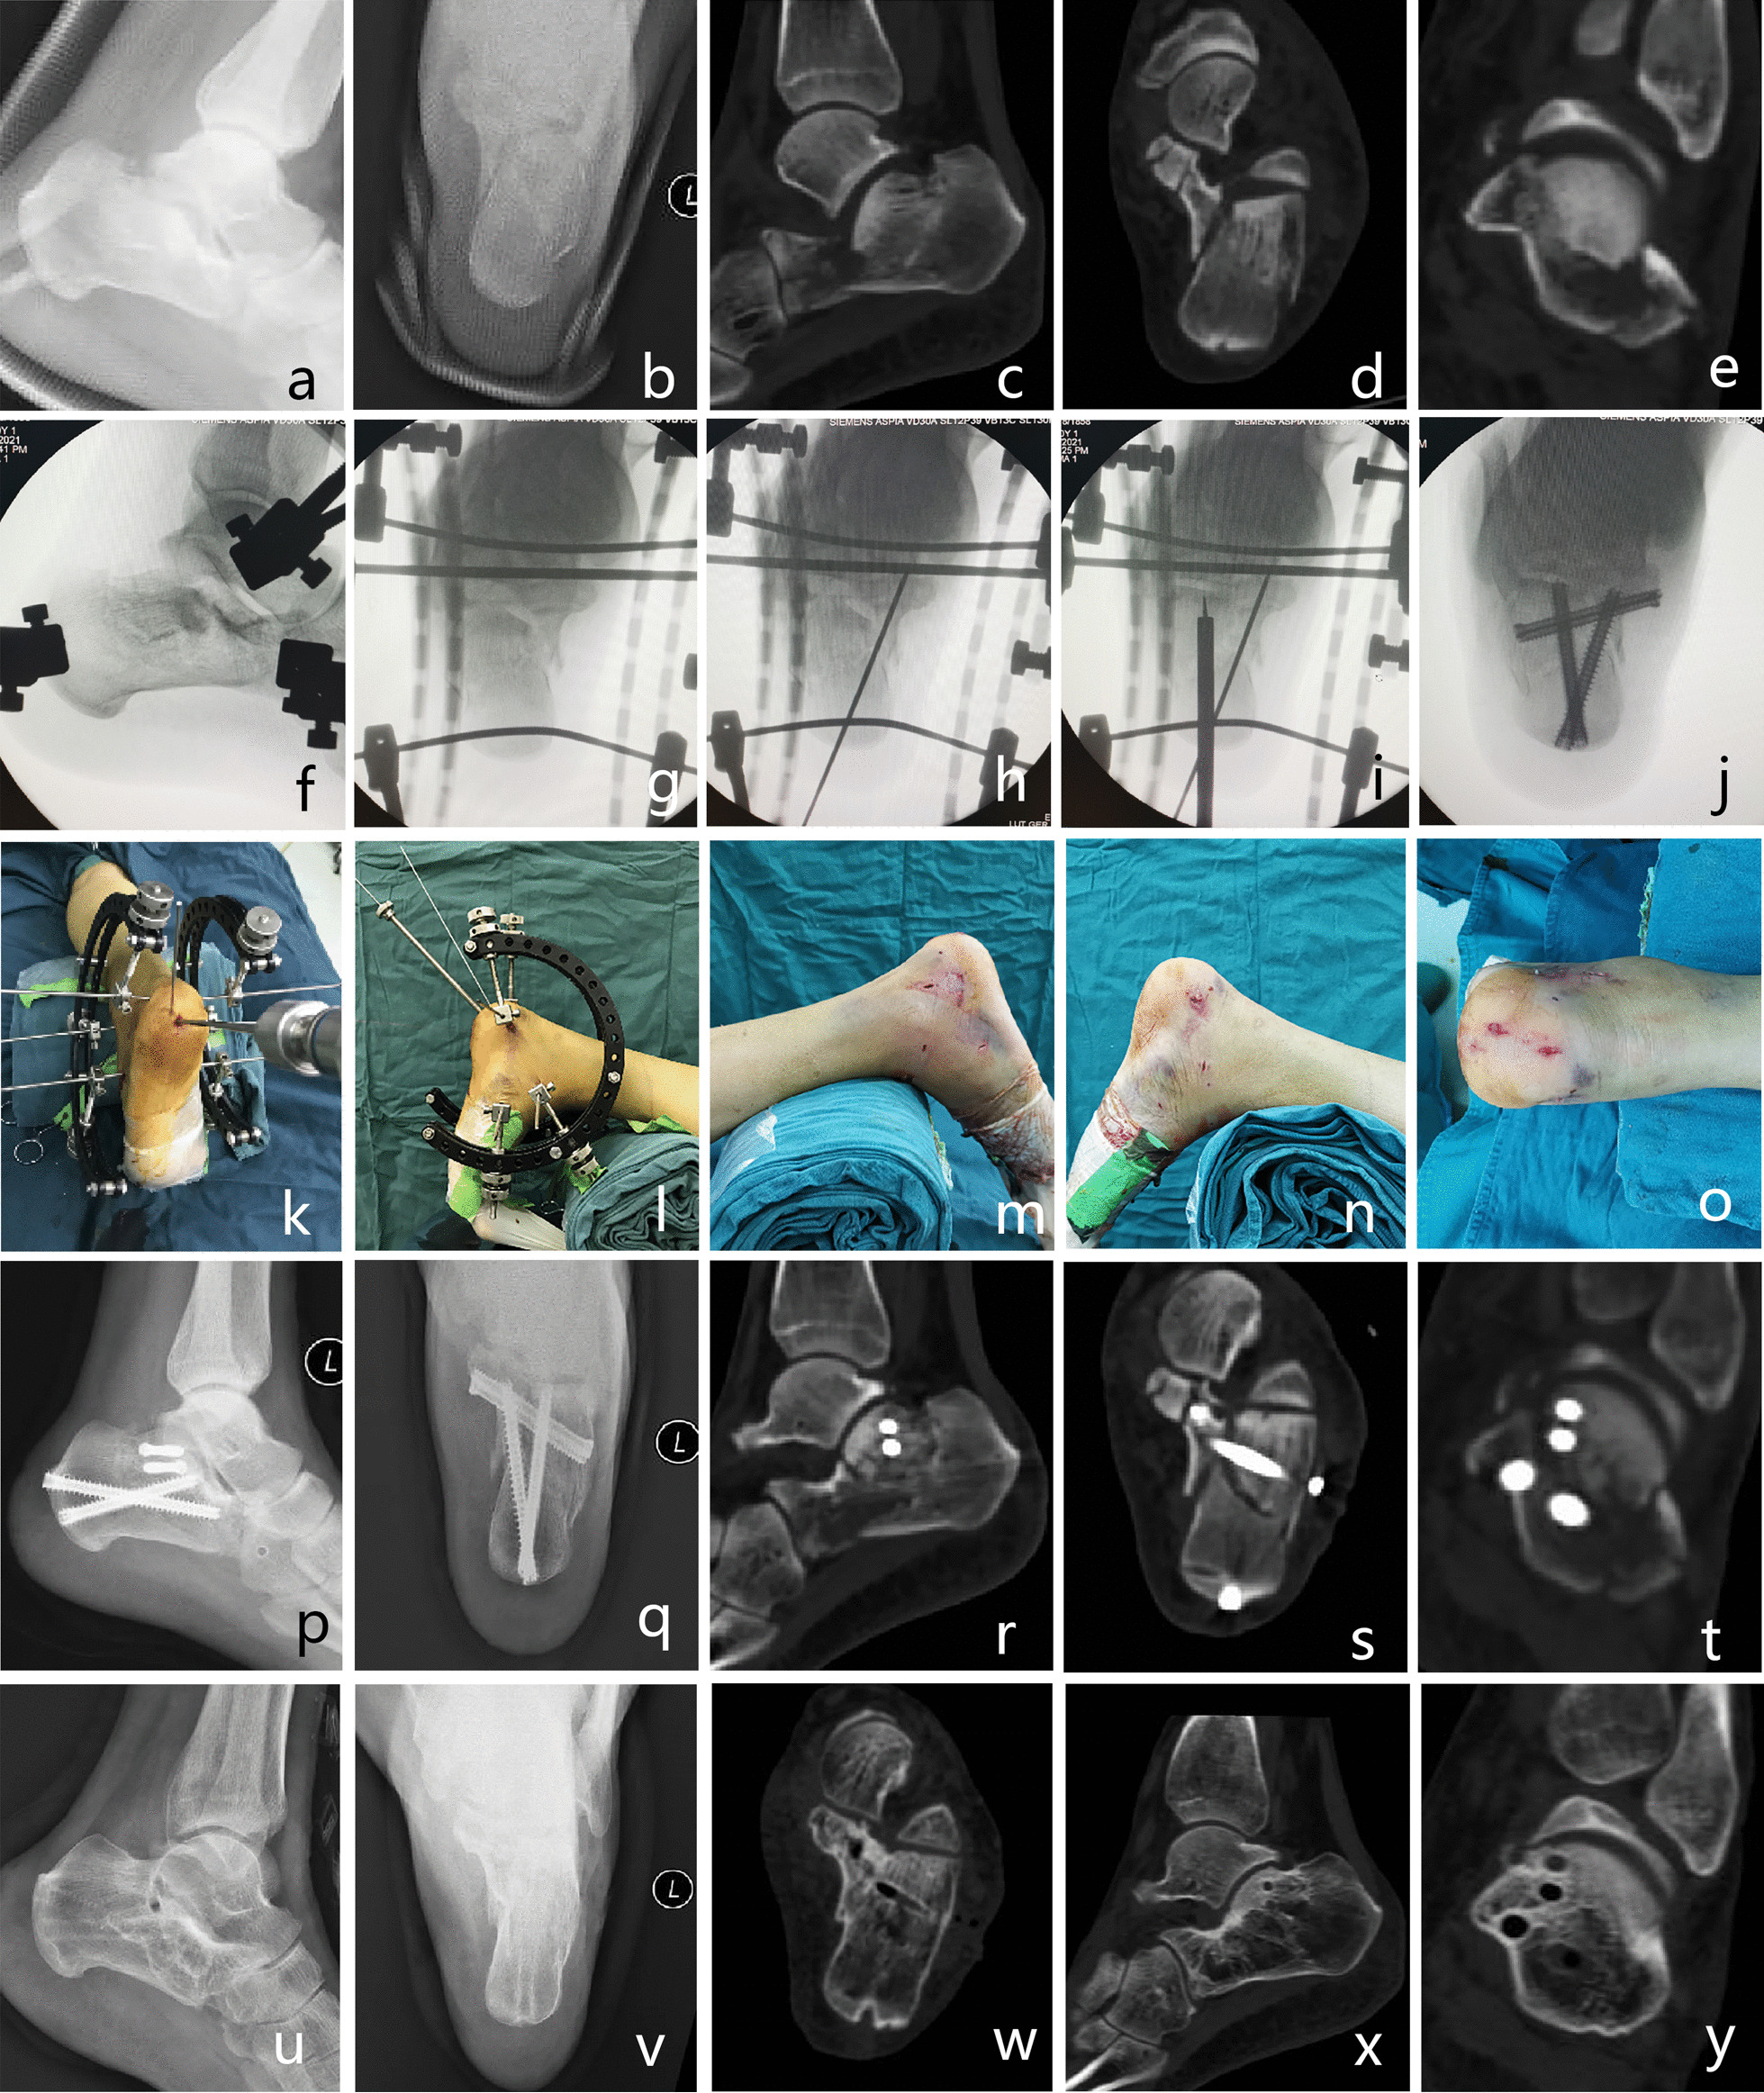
A 32-year-old male patient suffered left displaced intra-articular calcaneal fracture caused by a fall from a height, Essex-Lopresti classification (joint compression type), Sanders classification (II C). Preoperative lateral (a) and axial (b) X-rays, axial (c), sagittal (d) and coronal (e) CT views showed significantly decreased calcaneal height, Bohler angle and Gissanes angle, significantly increased calcaneal width and significantly collapse of the subtalar articular surface. Intraoperative fluoroscopy (f–j) showed the surgical procedures: (1) restored the morphological of the calcaneus by the TCDR (f, g), (2) temporarily fixed the medial calcaneus column with K-wire (h), (3) reduced the articular surface fracture fragments (i)and (4) percutaneously fixed by cannulated screws (j). Clinical photograph showed the percutaneous prying and jacking reduction technique (k, l) and the surgical incision (m–o). Immediate postoperative lateral (p) and axial (q) X-rays, axial (r), sagittal (s) and coronal (t) CT views showed ideal reduction of the calcaneal height/width, subtalar articular surface, Bohler angle and Gissanes angle. 14 months postoperative lateral (u) and axial (v) X-rays, axial (w), sagittal (x) and coronal (y) CT views showed fracture has healed without reduction lose
